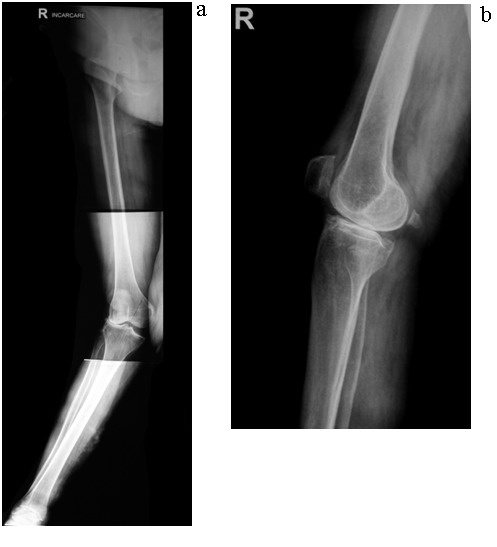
Preoperative long leg X-ray examination of the knee in standing position;AP view (a) and lateral view (b)
